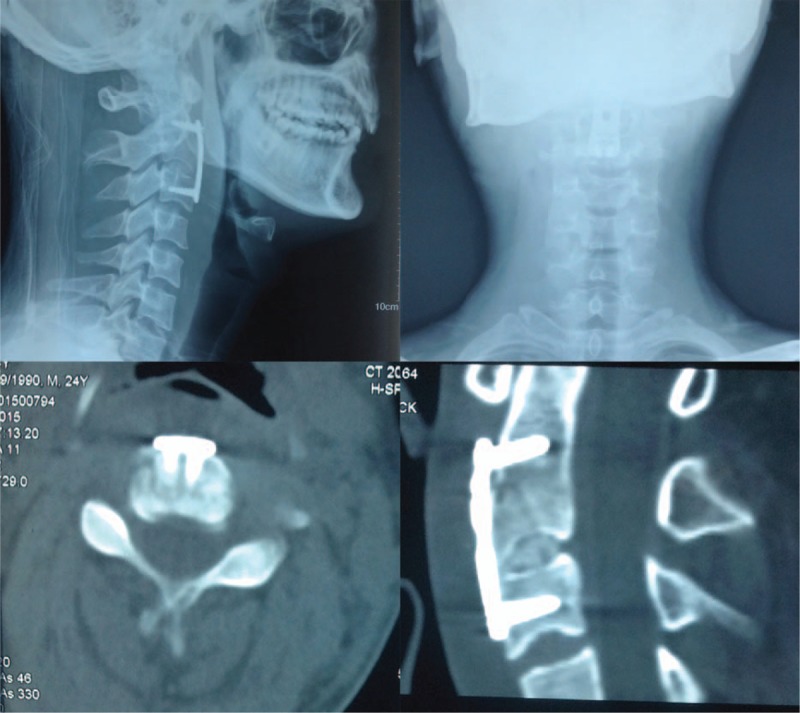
One day postoperative x-ray and CT scan showed a good position of the huge tear drop fracture fragment, three cortical iliac bones, and the anterior cervical plate. CT = computed tomography.
